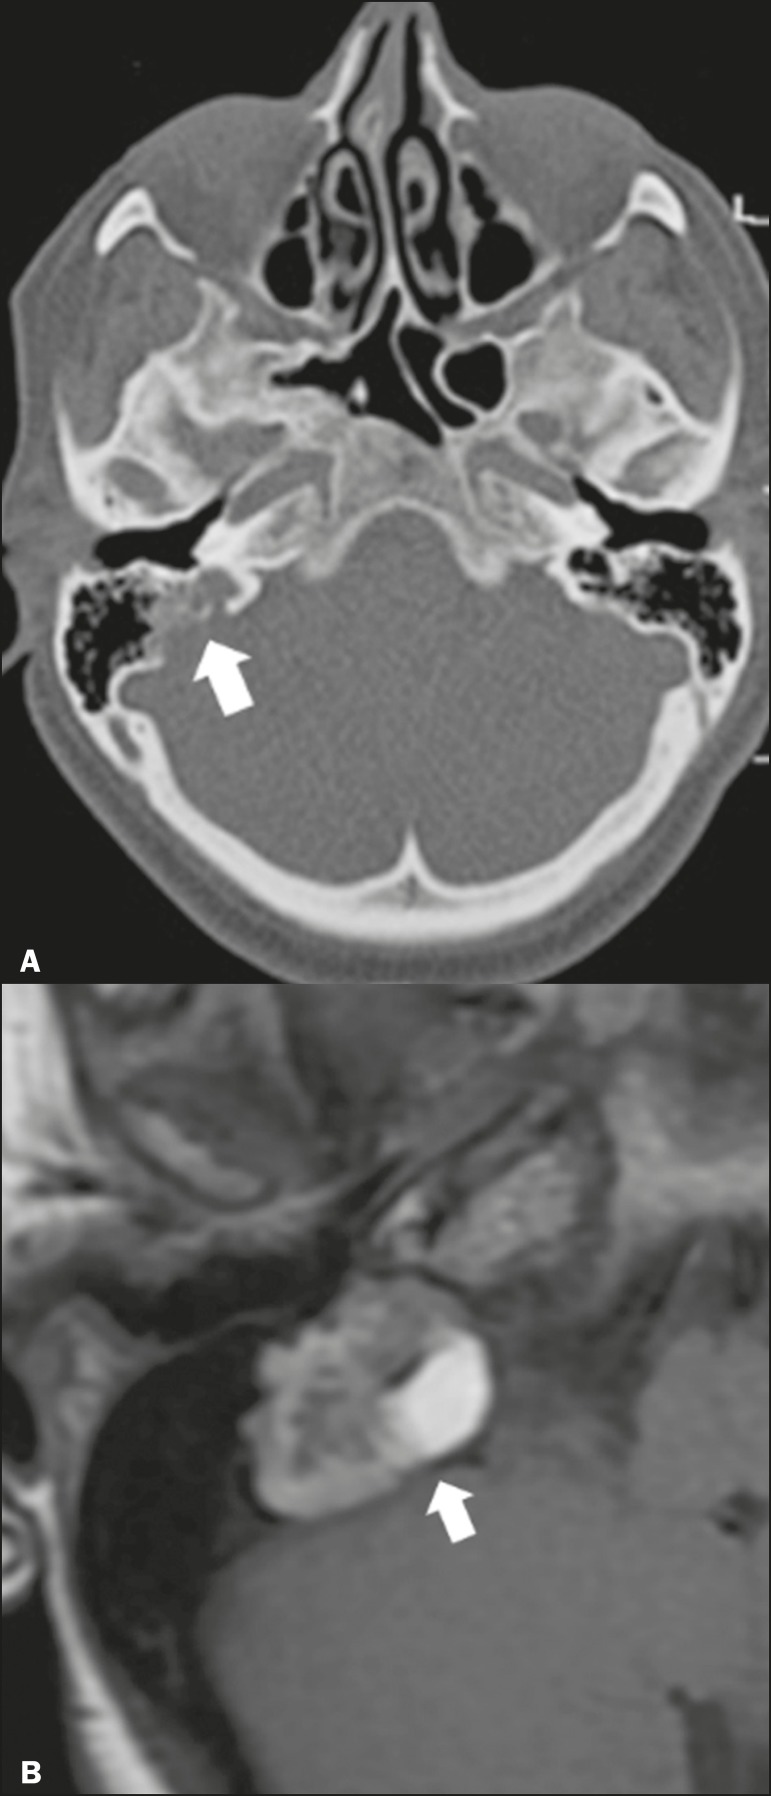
Endolymphatic sac tumor. A: Axial CT scan, with bone
